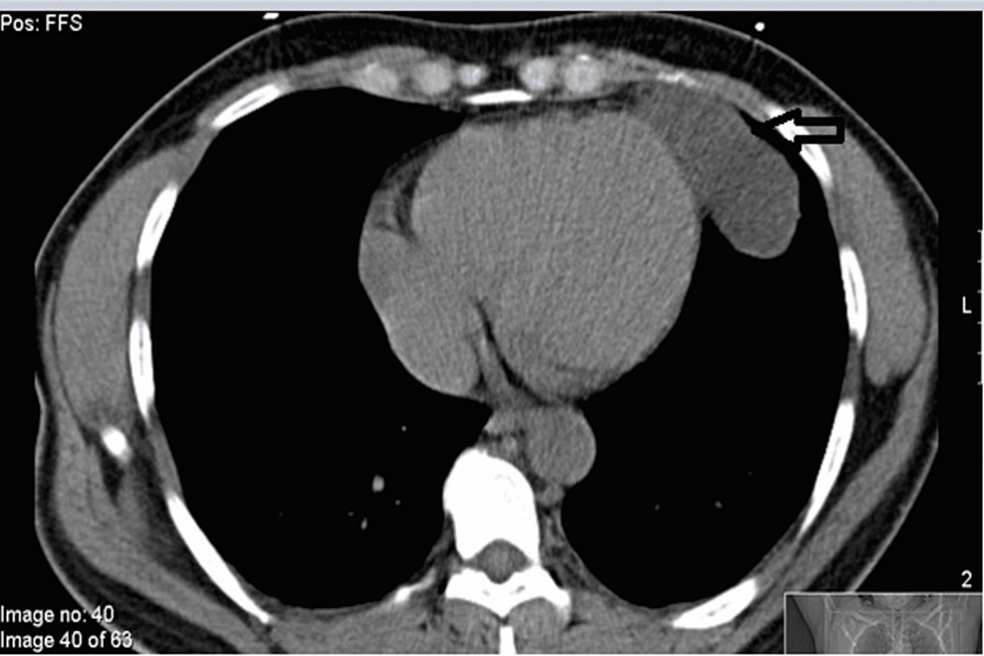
CT chest showing pericardial cyst measuring 8.1 x 4.2 cm (black arrow)
